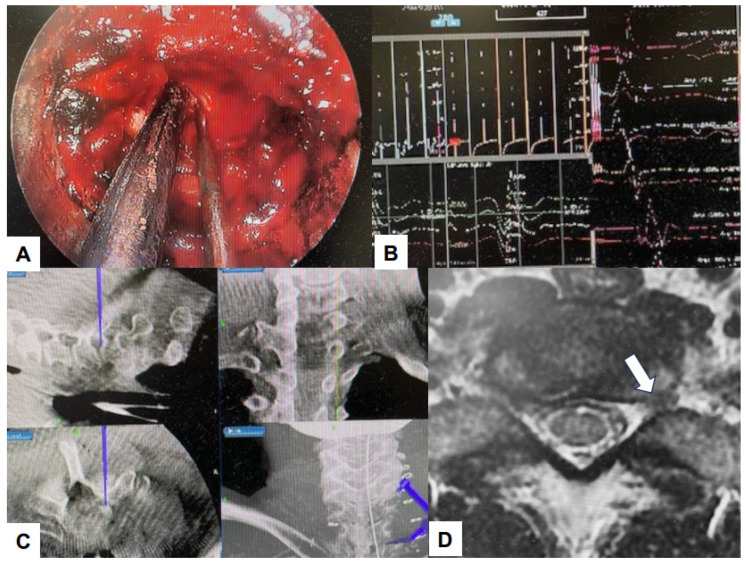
C arm free cervical endoscopic keyhole foraminotomy. (A) Endoscopy image, (B) Neuromonitoring, (C) Navigation monitor, (D) MRI. A white arrow shows cervical disc herniation.
